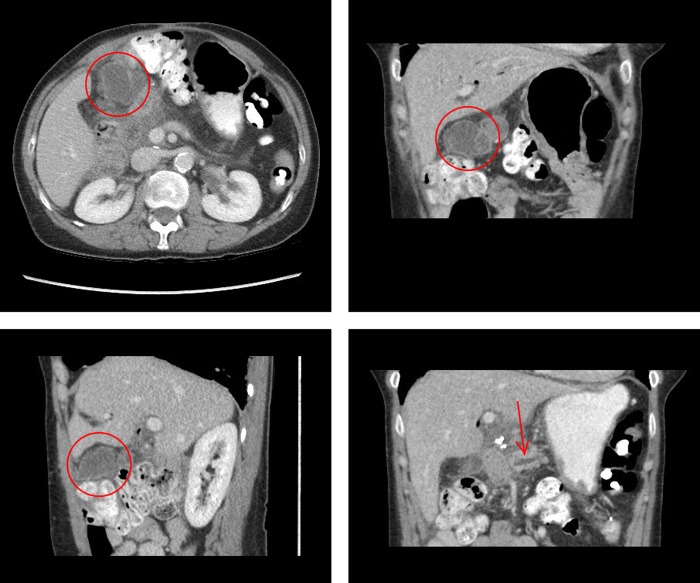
Computed tomography scan identifying a 4.6 × 3 cm collection near the pancreaticojejunostomy (red circle) as well as a prominent proximal pancreatic duct to 4 mm (arrow).
